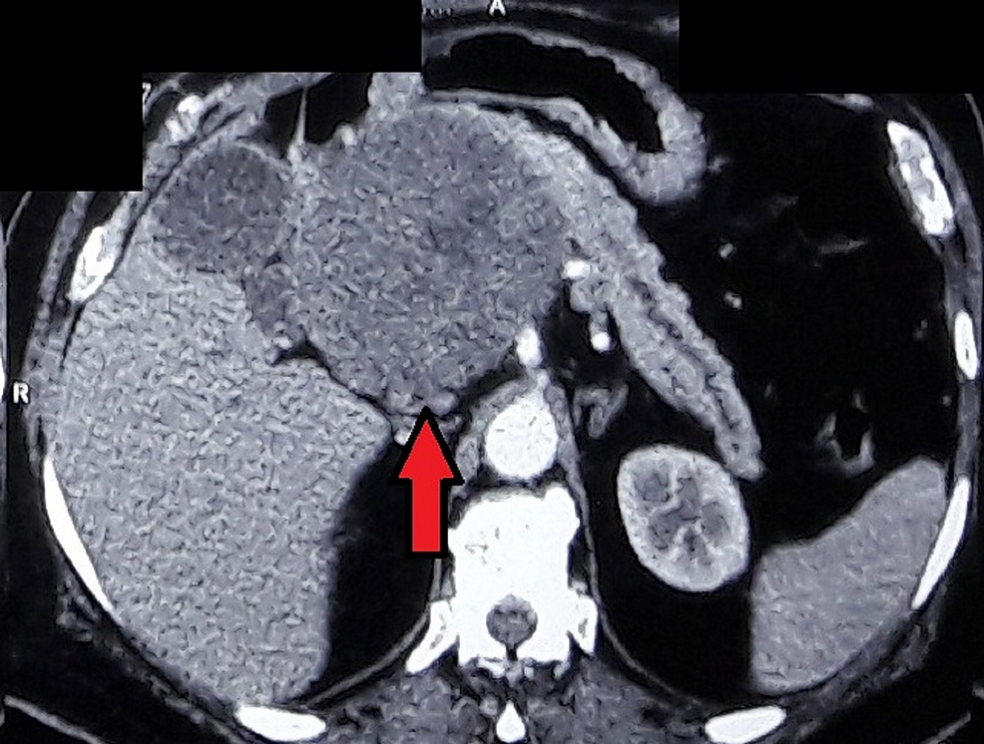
Contrast-enhanced computed tomography of the abdomen showing a 15 × 13 × 10-cm mass (red arrow) in the pancreatic head accompanied with a dilated pancreatic duct.
